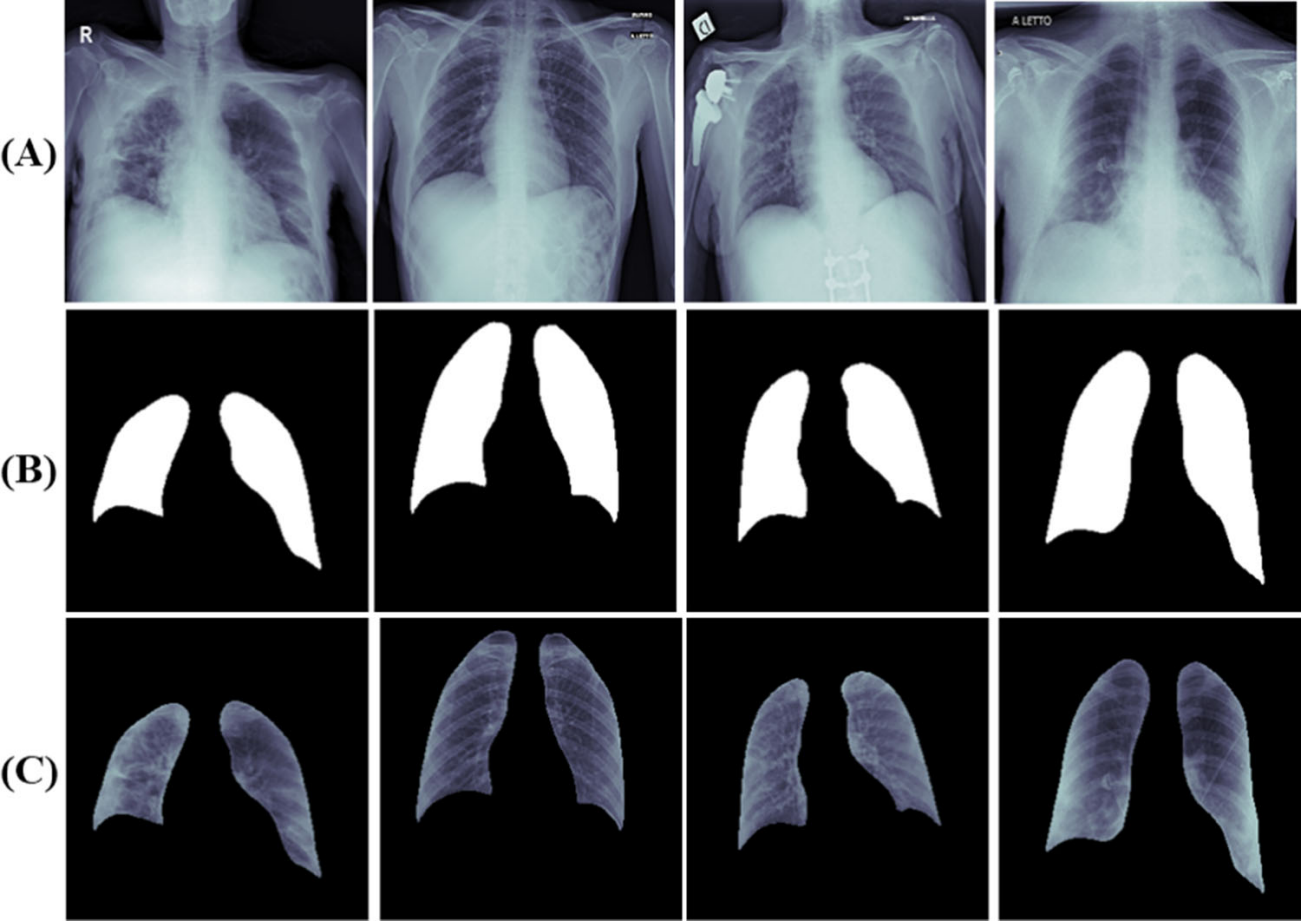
Samples X-ray images from the study dataset (A), generated masks by the best performing densenet121 FPN model (B) and corresponding segmented lung (C)
